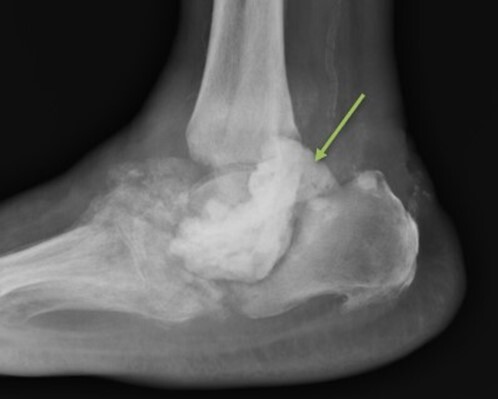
Case 3 sagittal foot radiograph demonstrating post-operative changes, including the placement of an antifungal cement spacer (arrow).
